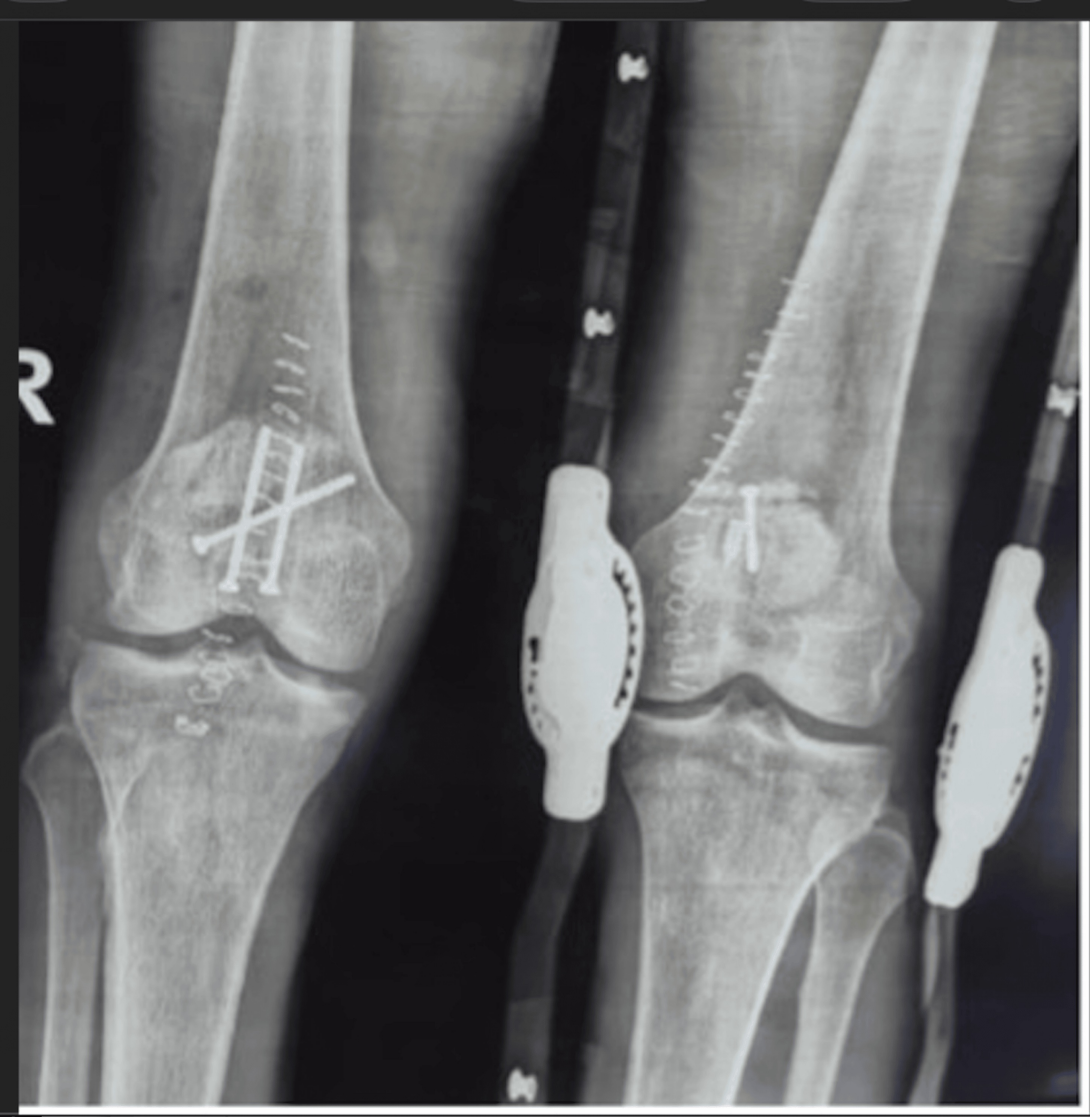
Radiographs of bilateral patellae (anteroposterior views) showing fixed patellar fractures
